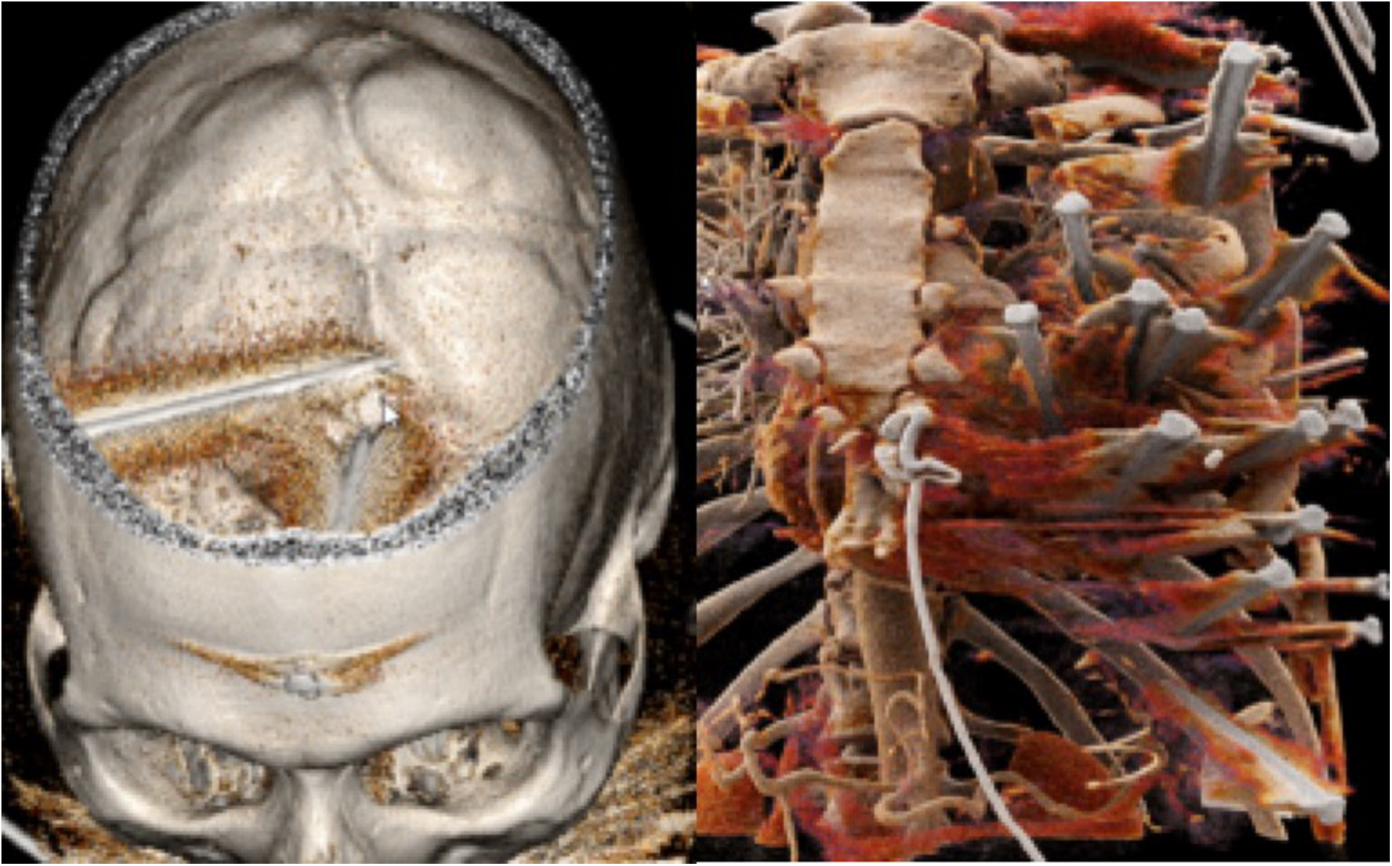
3d reconstruction of the CT-scan showing nails in the head (left) and those penetrating into the heart, the left lung and the stomach (right).
